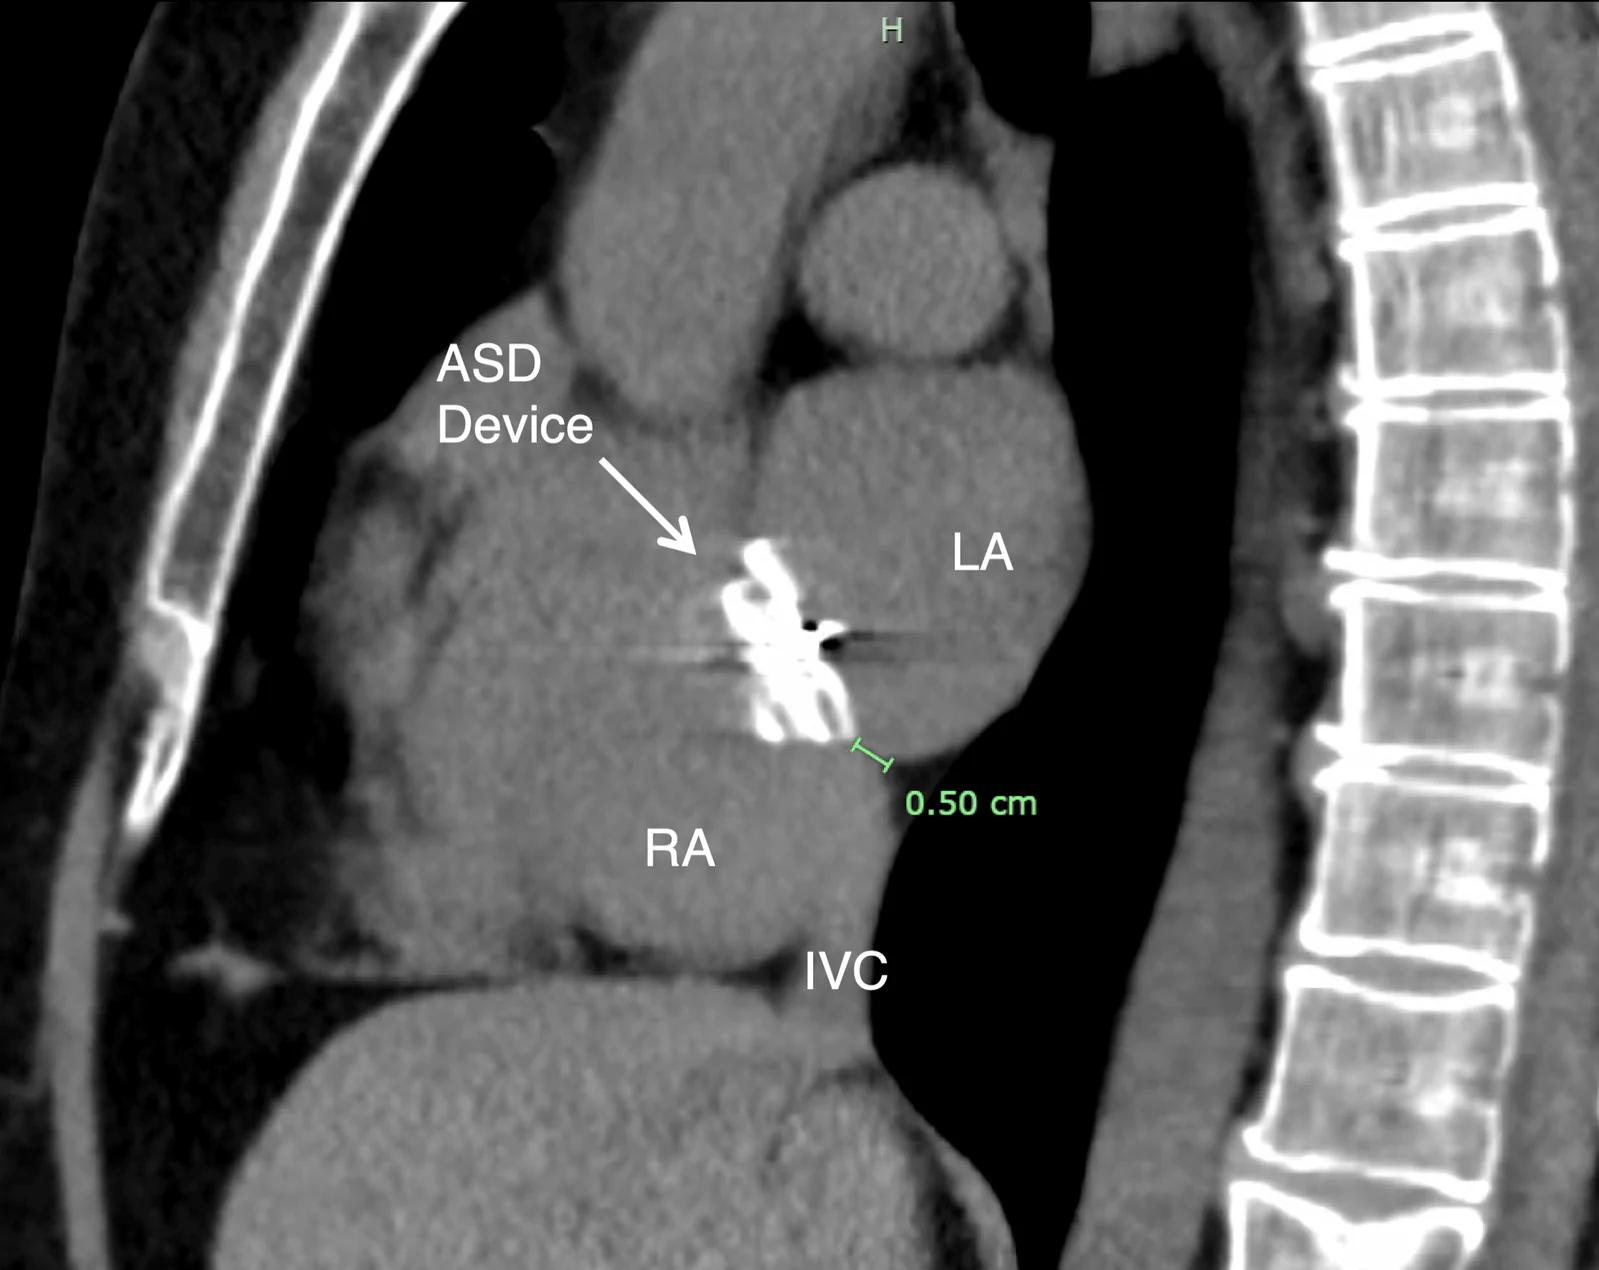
Preprocedural imaging - cardiac computed tomography Cardiac computed tomography demonstrating a limited (~5 mm) native septal tissue window inferior to the atrial septal defect (ASD) closure device. ASD: atrial septal defect; IVC: inferior vena cava; LA: left atrium; LAA: left atrial appendage; RA: right atrium.
